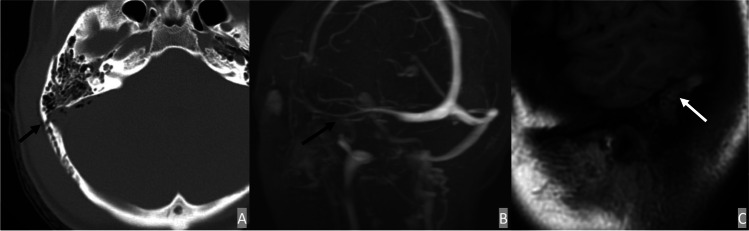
Venous sinus thrombosis from fracture of sigmoid groove. A 57-year-old male presented with a fracture along the right temporal bone with resulting venous sinus thrombosis. Axial CT head (A) demonstrates fracture along the tympanic portion of the right temporal bone with resultant pneumocephalus. 3D reconstructed image of MRV (B) demonstrates a focal area of filling defect in the right transverse-sigmoid sinus, compatible with venous sinus thrombosis. Sagittal T1 image (C) shows a focal thrombus with intrinsic T1 hyperintensity
